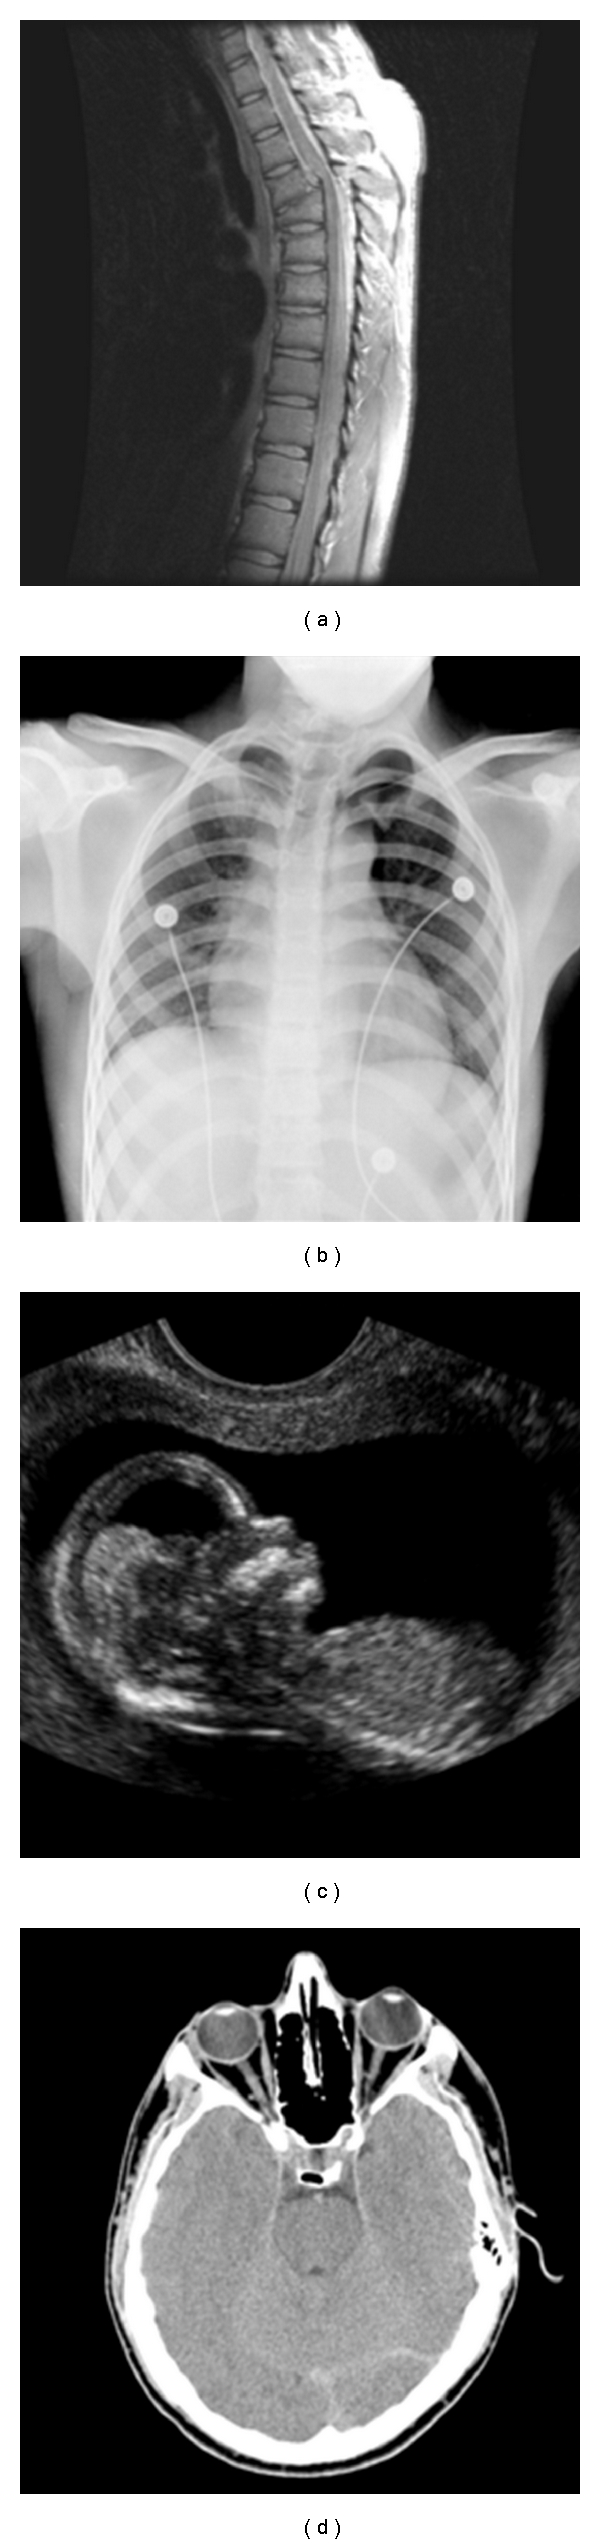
Test images; (a) Spine (MRI), (b) Chest (X-ray), (c) Fetus (ultrasonic), and (d) Head (CT).
